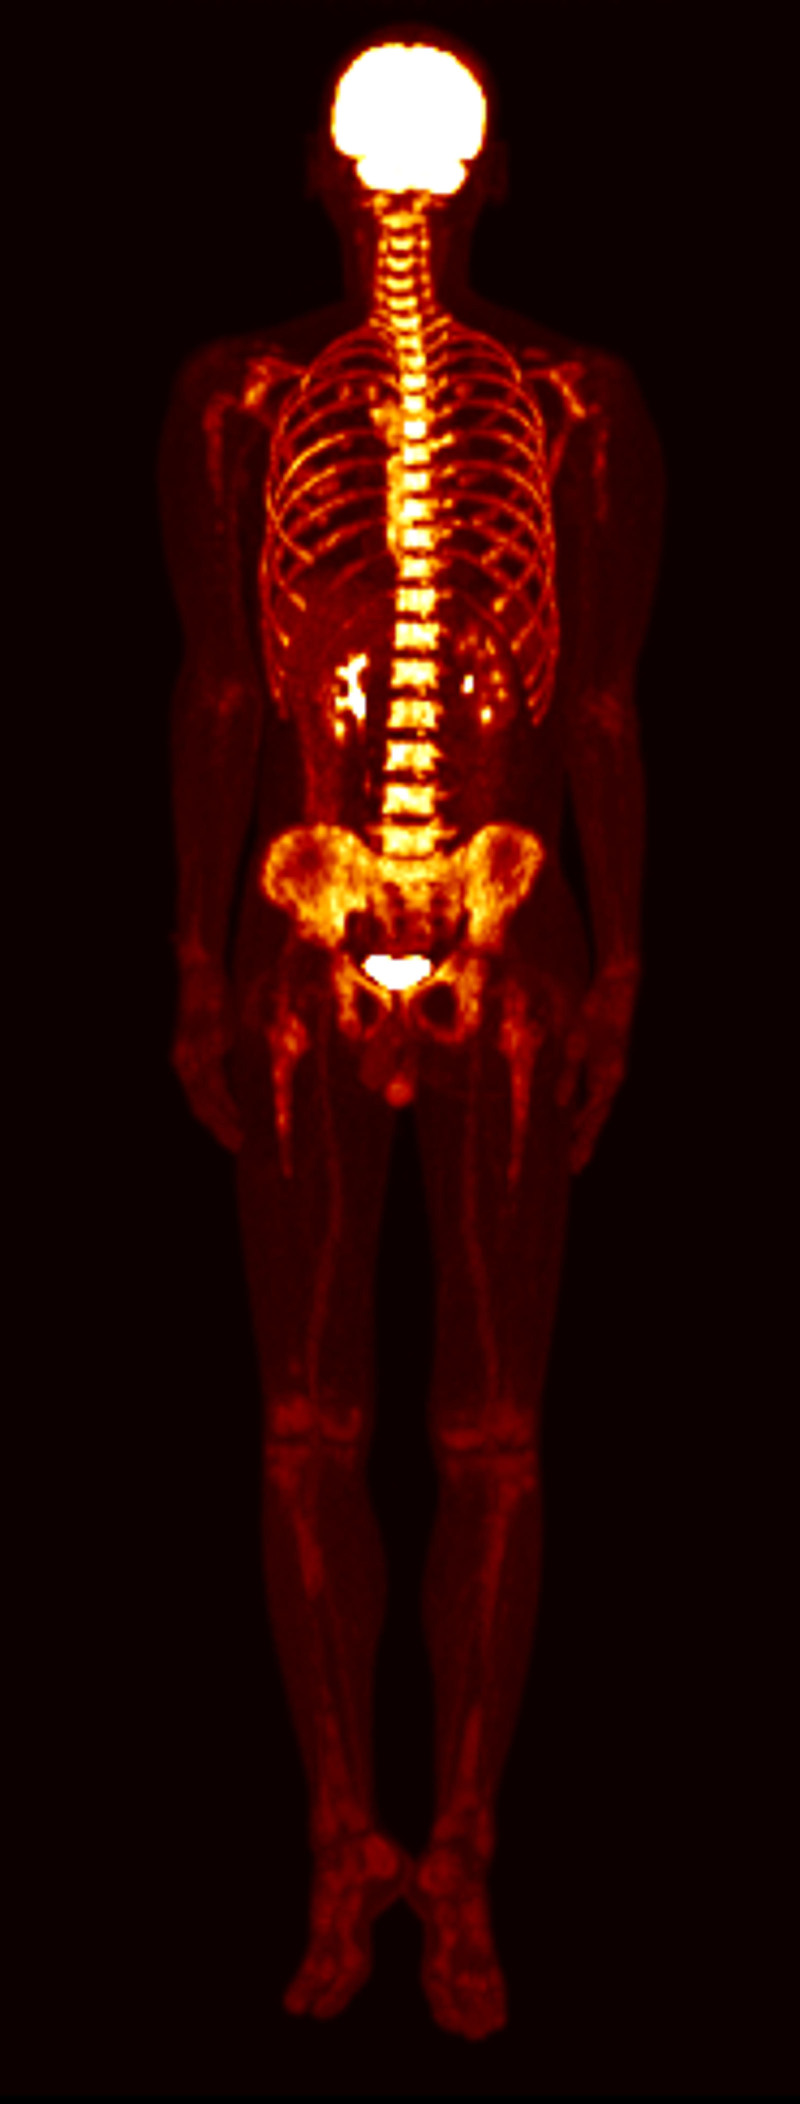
Follow-up FDG-PET Scan. No residual lesions in body. Probable reactive bone marrow change. FDG-PET = fluorodeoxyglucose-positron emission tomography.
